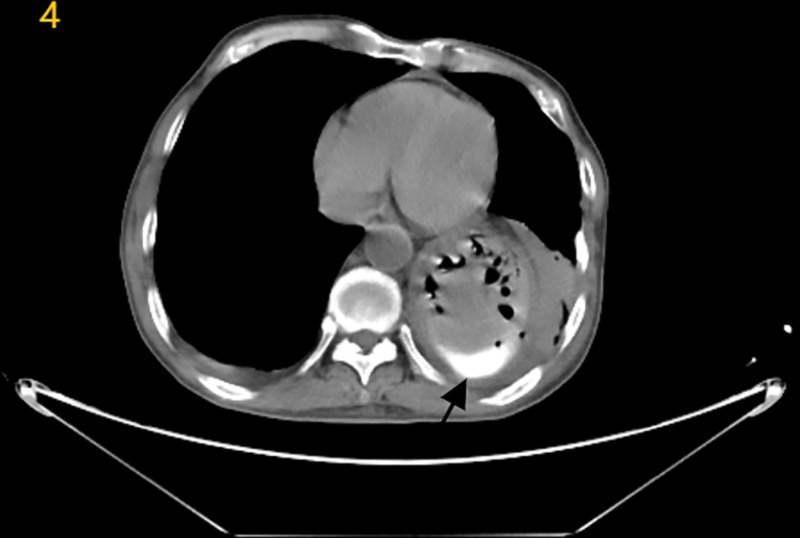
Follow-up CT on postoperative day 2 (arrows: irregular hyperdense material in the gastric lumen, consistent with residual contrast medium from the ruptured pseudoaneurysm).
